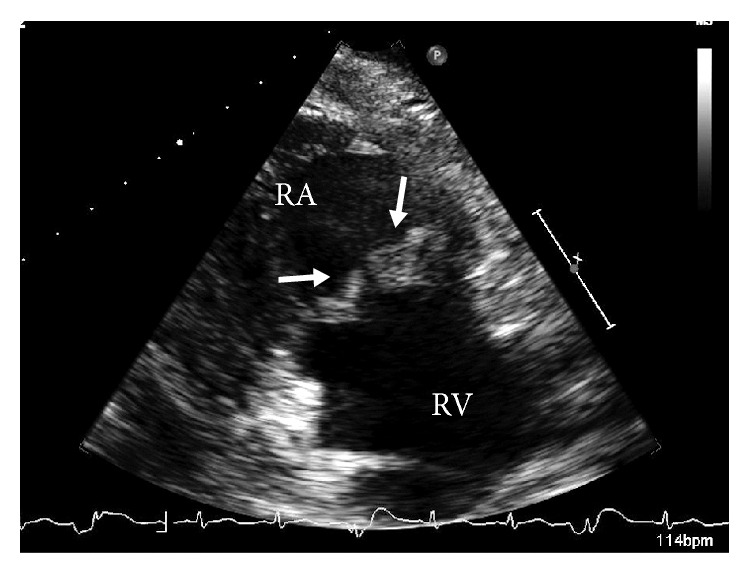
Transthoracic echocardiograph. Extensive bacterial vegetations are observed on the tricuspid (arrows) valves. RA: right atrium, RV: right ventricle.
